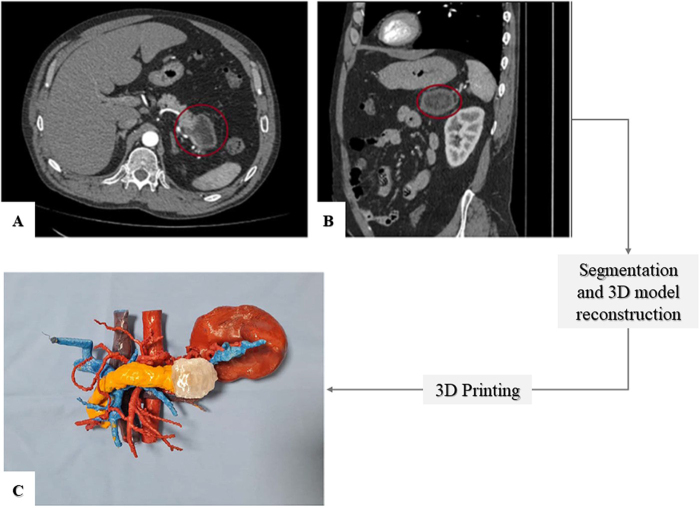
(A) Axial CT scan and (B) sagittal CT scan of a pancreatic cancer patient. Organs; (C) 3D printed model of patient’s anatomy. Image modified from^[38]^.
